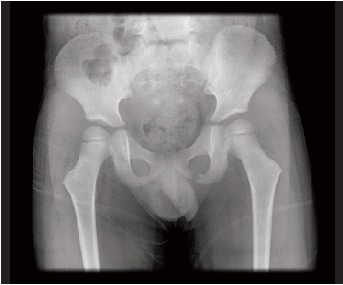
Fig. 1b: Plain frogview radiograph of the hips. F1b:
